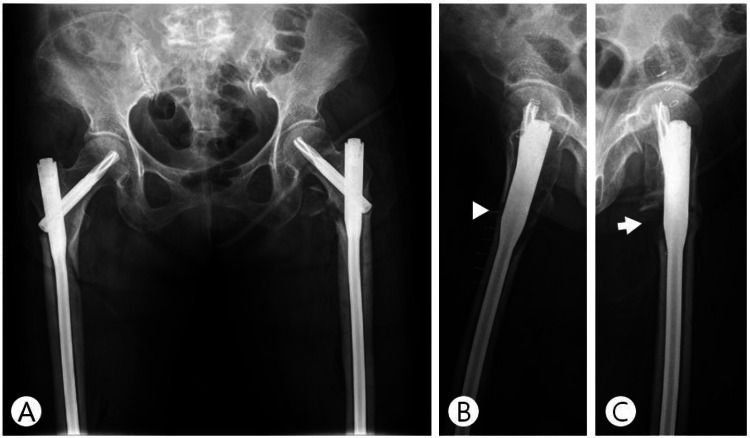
Postoperative radiographs of both femur subtrochanteric atypical fractures treated with long proximal femoral nail antirotation II. The images show (A) anteroposterior radiograph of both hip joints, (B) right lateral radiograph shows gap between fracture fragments (arrowhead), and (C) left lateral radiograph shows bone defect due to displaced lesser trochanteric fracture fragment (arrow).
